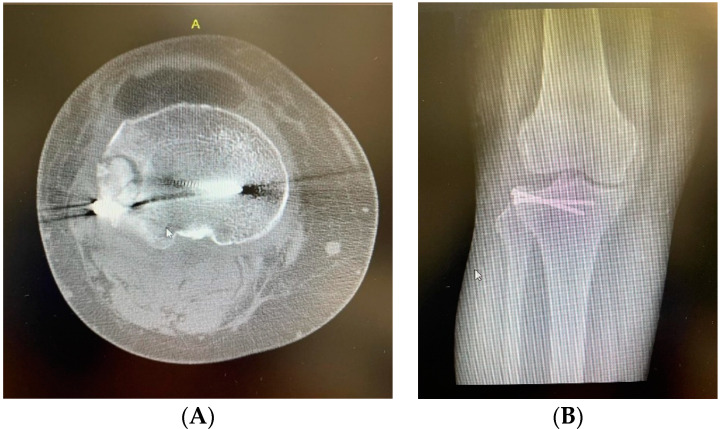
Post-operative CT scan of the right knee. (A) Post-operative AP CT scan of the right knee showing the reduction and fixation of the fracture after augmentation with sticky bone. (B) Post-operative AP plain radiograph of the right knee showing the reduction and fixation of the fracture after augmentation with sticky bone.
